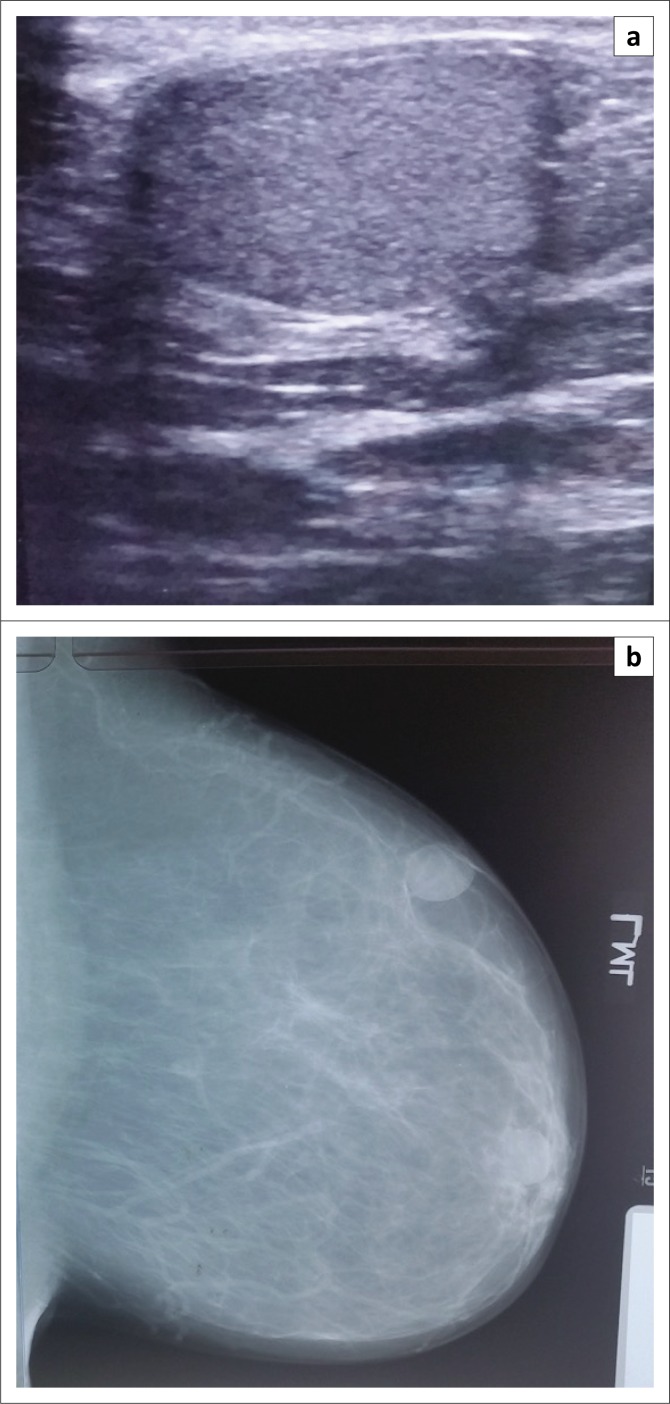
A palpable left breast lump in a 48-year-old woman. She had a breast trauma 3 years prior to presentation: (a) Ultrasound of the left breast showed a well-defined hyperechoic mass and (b) left medio-lateral oblique mammogram showing a mass with a calcified (egg shell) rim. At the patient’s request, she had a biopsy, which confirmed an oil cyst (from post-traumatic fat necrosis).
